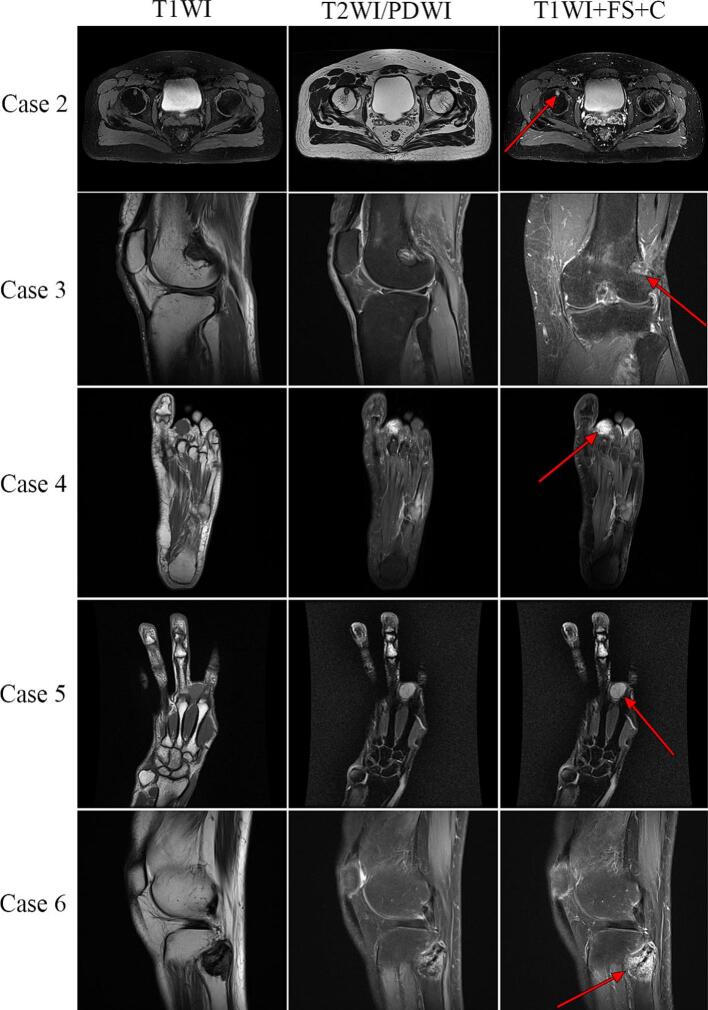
Magnetic Resonance Imaging (MRI). The tumors exhibited low signal intensity on T1-weighted imaging (T1WI), while on T2-weighted imaging (T2WI), they appeared with intermediate to high signal intensity (compared to the surrounding normal muscle tissue). On proton density-weighted imaging (PDWI), the tumors showed high signal intensity. Contrast-enhanced scans demonstrated either homogeneous enhancement (Case 2, 4, and 5) or heterogeneous enhancement (Case 3 and 6).The tumor sizes were 11 × 10 × 9 mm, 18 × 16 × 15 mm, 19 × 15 × 15 mm, 17 × 16 × 15 mm, and 30 × 30 × 25 mm, respectively.
